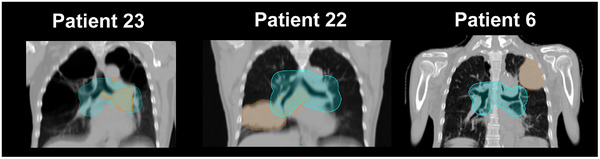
Representative planning computed tomography (CT) images of selected patients. Planning CT images of Patients 23, 22, and 6 are shown in comparable coronal planes. Areas shaded in orange represent the total gross tumor volume (GTV), and those in light blue represent the region within 2 cm of the proximal bronchial tree. The regions where orange and light blue overlap delineate the proximal GTV. Images from three representative patients are shown, displayed in comparable coronal planes.
